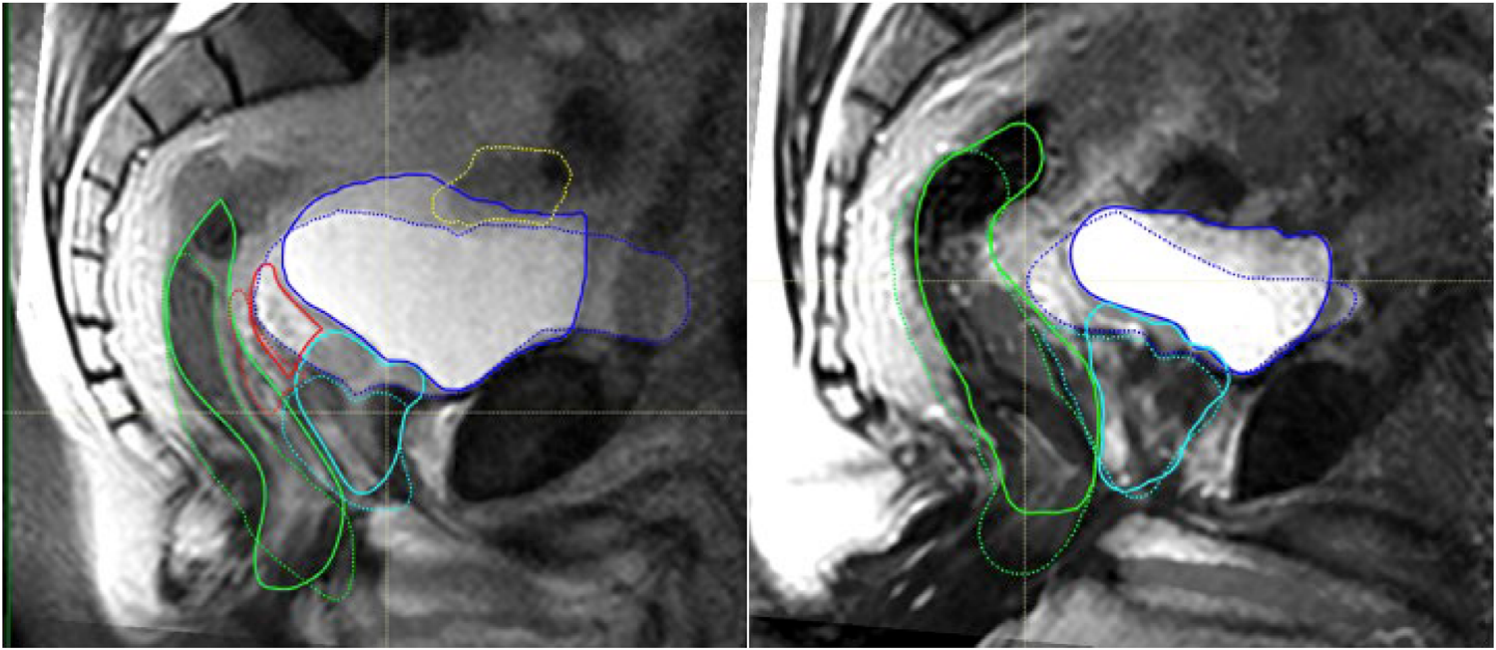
Midline sagittal MRI images for two volunteers in the supine and upright full bladder conditions. Superimposed on the images are contour lines delineating the organs in the supine (solid lines) and upright (dashed lines) positions. The following organs are also shown in both panels—rectum (green), prostate (light blue), bladder (blue) and small bowel (yellow—left panel only).
